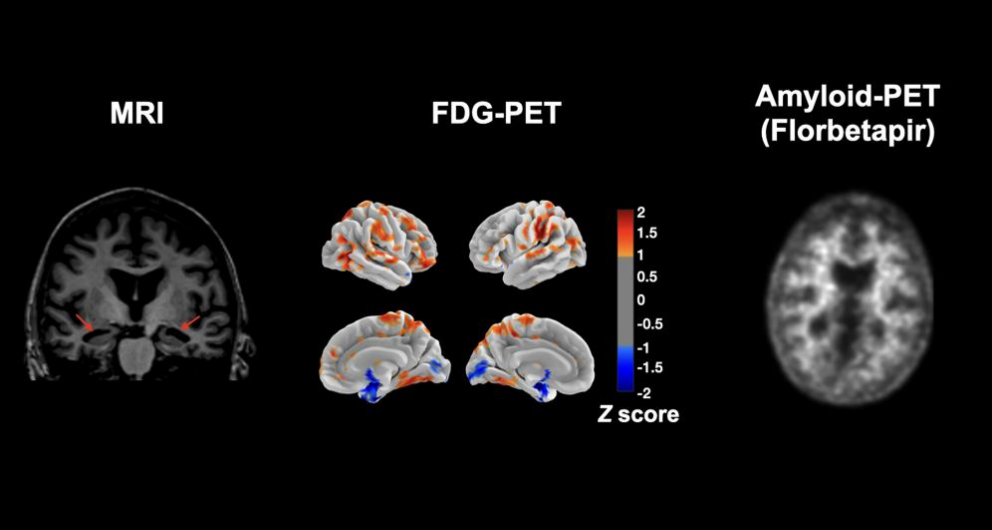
Case example of a prospective use of the LANS criteria in clinical settings. This patient was seen at Mayo Clinic, Rochester, to determine eligibility for an anti-amyloid monoclonal antibody therapy, and the LANS criteria were internally defined at the time of evaluation. The criteria were operationalized using clinical judgement of available data including visual reads of neuroimaging as is the current standard in clinical practice. This patient was a female over the age of 75 with a history of memory problems for several years. She lived alone, managed her instrumental activities of daily living independently and was still working part-time. Neuropsychological testing revealed moderate to severe impairment on measures of delayed recall and fragility of salient semantic knowledge (i.e. she could not recall details about the events occurring on 11 September 2001; she named another building than the World Trade Center and did not know how many buildings were hit). Performance was also mildly low on a task of object naming. The remainder of the assessment (i.e. global cognition, executive functions, visuospatial processing and language) was within or above expectations from a normative standpoint. The profile was consistent with single-domain amnestic mild cognitive impairment. MRI revealed disproportionate bilateral hippocampal atrophy, and she was assigned a moderate likelihood LANS diagnosis. Subsequently, a brain FDG-PET revealed prominent temporo-limbic and milder inferior frontal hypometabolism in the absence of a neocortical degenerative pattern by visual read. Amyloid-PET was read as negative. She thus met all core, standard and advanced criteria for LANS, corresponding to the highest likelihood. She did not meet criteria for an anti-amyloid monoclonal antibody therapy and was counselled about diagnosis, prognosis and most likely underlying aetiology.
